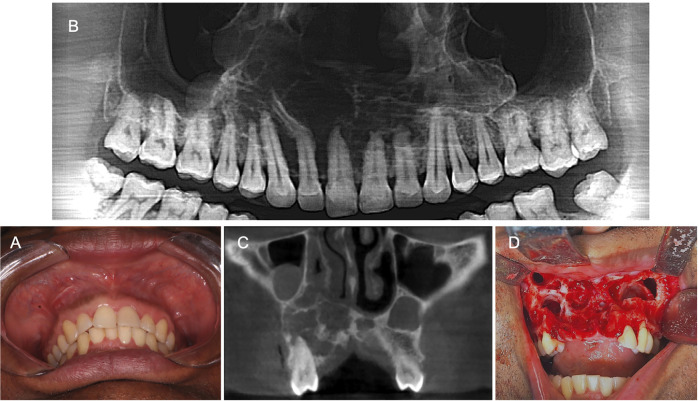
Case 3 clinical and tomographic findings. A, Intraoral examination showed no alterations. B, CBCT panoramic reconstruction demonstrating a multilocular image with sclerotic borders located in the anterior maxilla. C, CBCT scan showing teeth roots associated with the lesion and rupture of the lower cortical of the nasal cavity. D, After performing a total mucoperiosteal flap and removing the teeth in association with the lesion, observe the presence of multiple cystic spaces within the bone.
